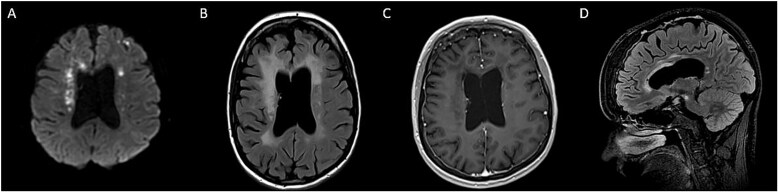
Brain MRI showing diffusion restriction (A) with T2 FLAIR confluent periventricular hyperintensity, ventricular enlargement due to frontoparietal atrophy (B) and atrophy of genu and anterior body of the corpus callosum (D) without enhancement (C).
